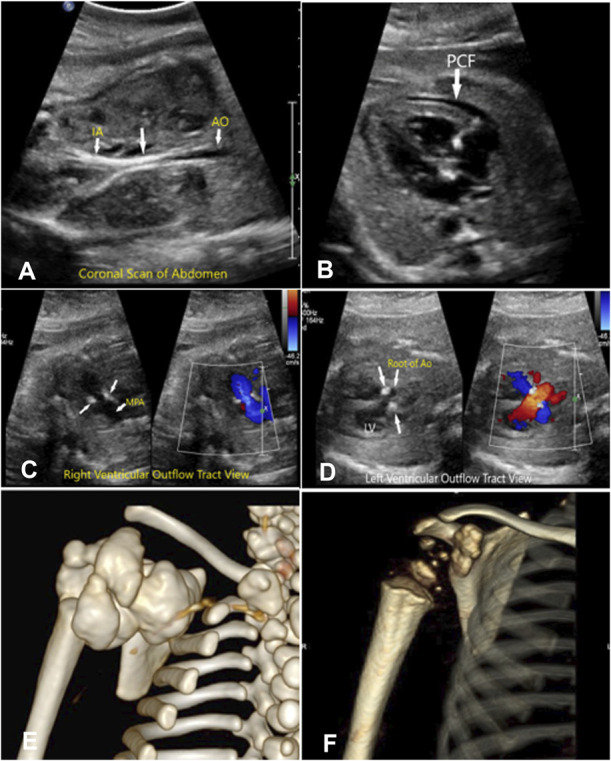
A. Coronal scan of the fetal abdomen shows the calcification (*arrows*) of the aorta (AO) and iliac (IA) arteries. B. Four-chamber view of the fetal heart showing pericardial effusion (PCF). C. Right ventricular outflow tract showing the calcification (*arrows*) of the main pulmonary artery (MPA). D. Left ventricular outflow tract showing the calcification (*arrows*) of the root of AO. E. Three-dimensional computed tomography image of the right shoulder at day 4. F. Three-dimensional computed tomography image of the right shoulder at age 20 months. LV, left ventricle.
